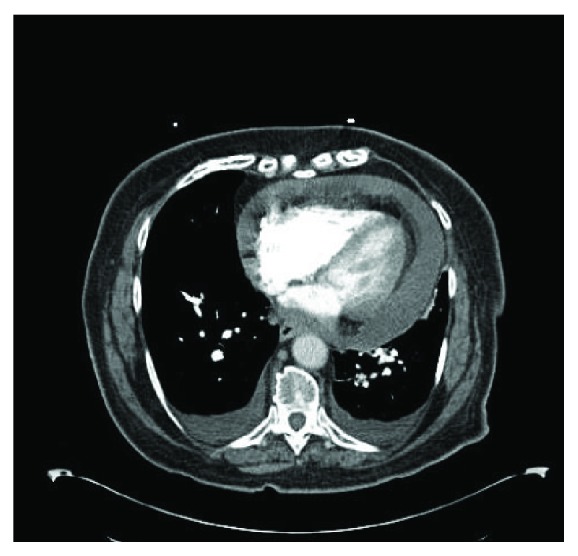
Computed tomography of the chest demonstrating pericardial effusion.
